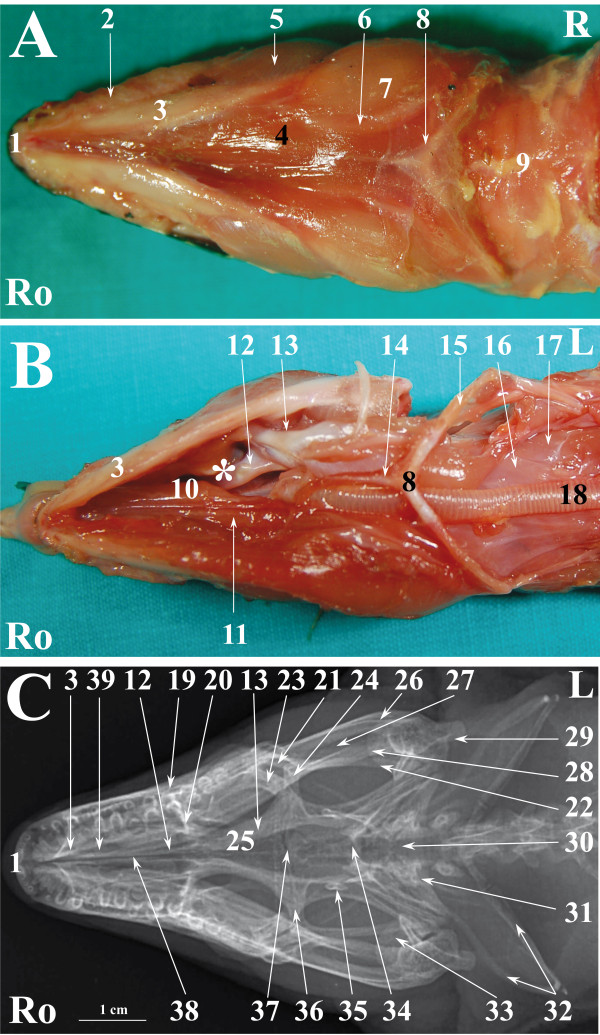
Normal radiographic anatomy of the head of the common tegu in VD projection.A. Ventral view in a superficial plane of stratigraphic dissection (only skin was removed) of the head of a tegu. B. Ventral view of stratigraphic dissection after removal of Musculus constritor colli and Musculus intermandibularis (lower part of the image) and a deeper plane of dissection (upper part of the image) of the head of a tegu (deeper plane of dissection is labelled with * on the image). C. VD radiographic projection of the head of a tegu. Ro is rostral, L is left.1. Premaxillary bone; 2. Infralabial glands; 3. Dentary bone; 4. Musculus intermandibularis; 5. Musculus adductor mandibulae externus superficialis; 6. Musculus hyoglossus; 7. Musculus pterygoideus; 8. Basihyal bone; 9. Musculus constrictor colli; 10. Tongue; 11. Oesophagus; 12. Palatine bone; 13. Pterygoid bone; 14. Entoglossal process of hyobranchial skeleton; 15. Ceratobranchial process of hyobranchial skeleton; 16. Musculus omohyoideus; 17. Musculus sternohyoideus; 18. Trachea; 19. Maxillary bone; 20. Prefrontal bone; 21. Jugal bone; 22. Squamosal bone; 23. Ectopterygoid bone; 24. Postorbital bone; 25. Frontal bone; 26. Surangular bone; 27. Adductor fossa; 28. Angular bone; 29. Articular bone; 30. Basioccipital + Supraoccipital bones; 31. Paraoccipital process of otooccipital bone; 32. Hyobranchial skeleton; 33. Quadrate bone; 34. Parietal bone; 35. Epipterygoid bone; 36. Postfrontal bone; 37. Parieto-frontal suture; 38. Interpterygoid vacuity; 39. Vomer bone.
